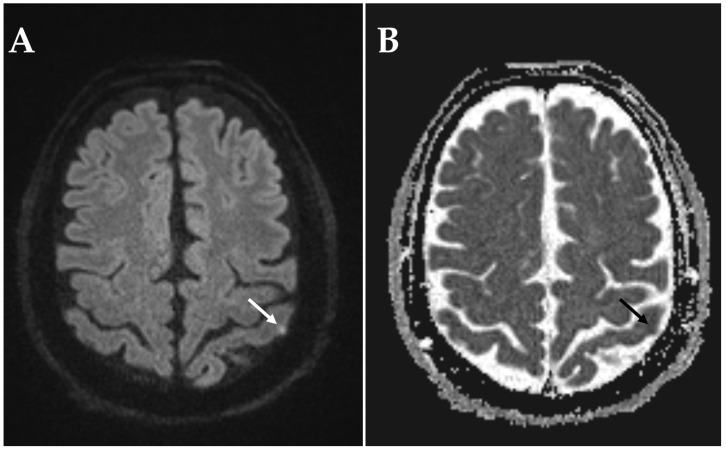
MRI sequences showing a small acute embolic infarction in a patient with NAION of the left-eye. MRI was performed on a 3 T scanner after three days following onset of visual impairment. (A) DWI reveals a small hyperintense cortical lesion in the territory of the left middle cerebral artery. (B) ADC with corresponding focal hypointense signal.
